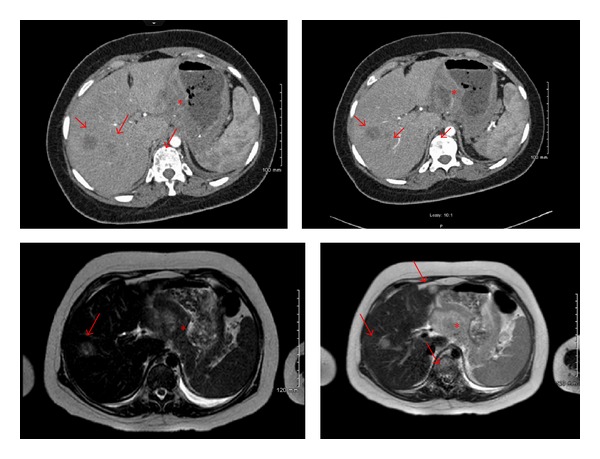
Incidental finding of thickening of stomach lining (asterisk), multiple liver and spinal metastases (arrows) found in the CT with contrast angiogram of the chest.
